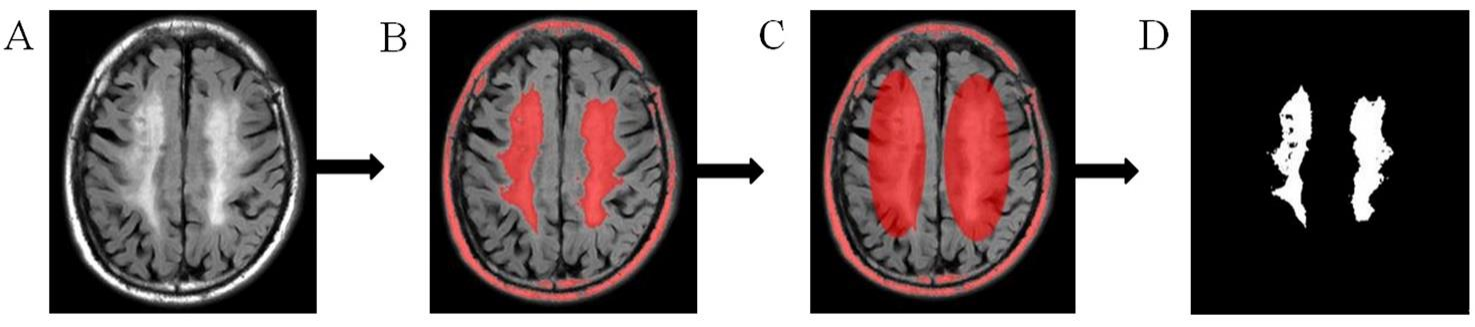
Quantitative steps of WMH volume. (A) Original FLAIR image. (B) The high signal area. (C) A sketch of the effective WMH area. (D) Extract of the effective WMH area.
